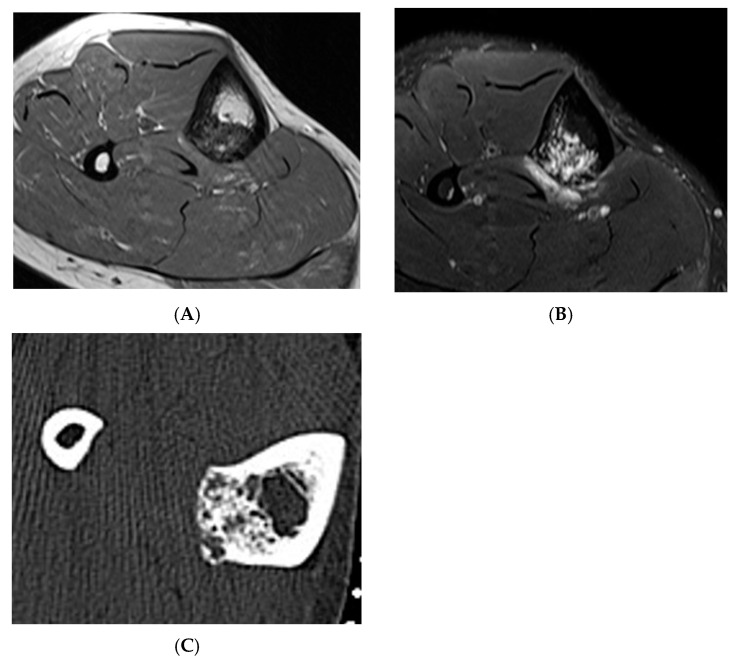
Haemangioma, tibia. (A) Axial T1W MRI. (B) Axial STIR MRI. (C) Axial CT demonstrating “honeycomb” pattern of intraosseous haemangioma. Low signal intensity on T1 with corresponding high SIR signal on STIR suggests the low fat content of the lesion as seen on CT with few interspersed areas of hypodensity in the lesion.
